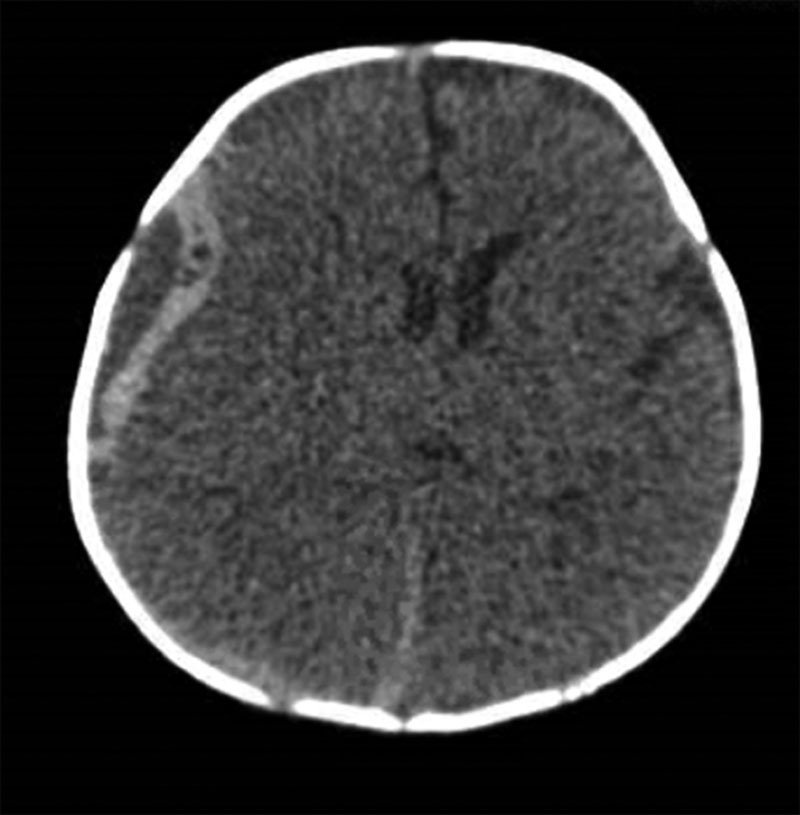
A non-contrast cranial CT showing hyperdense biconvex collection suggesting hematoma with midline displacement.
